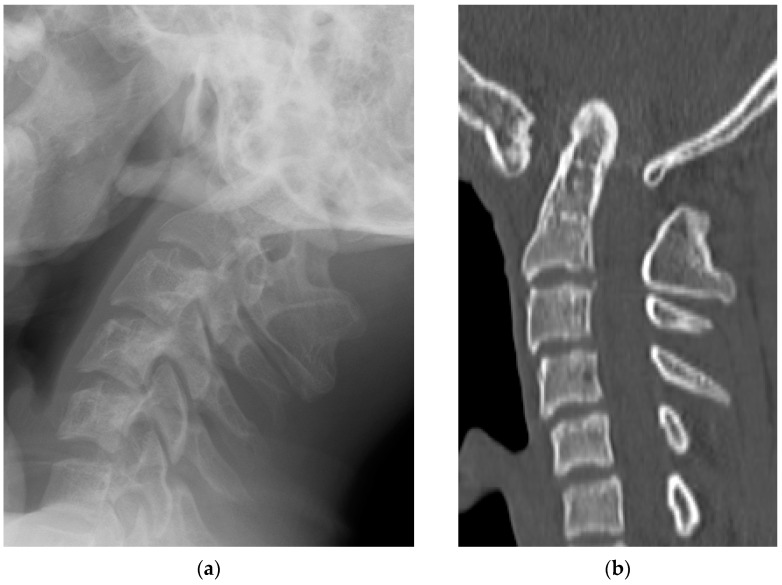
Preoperative radiographic images: (a) lateral X-ray view; (b) sagittal view of a computed tomography scan.
